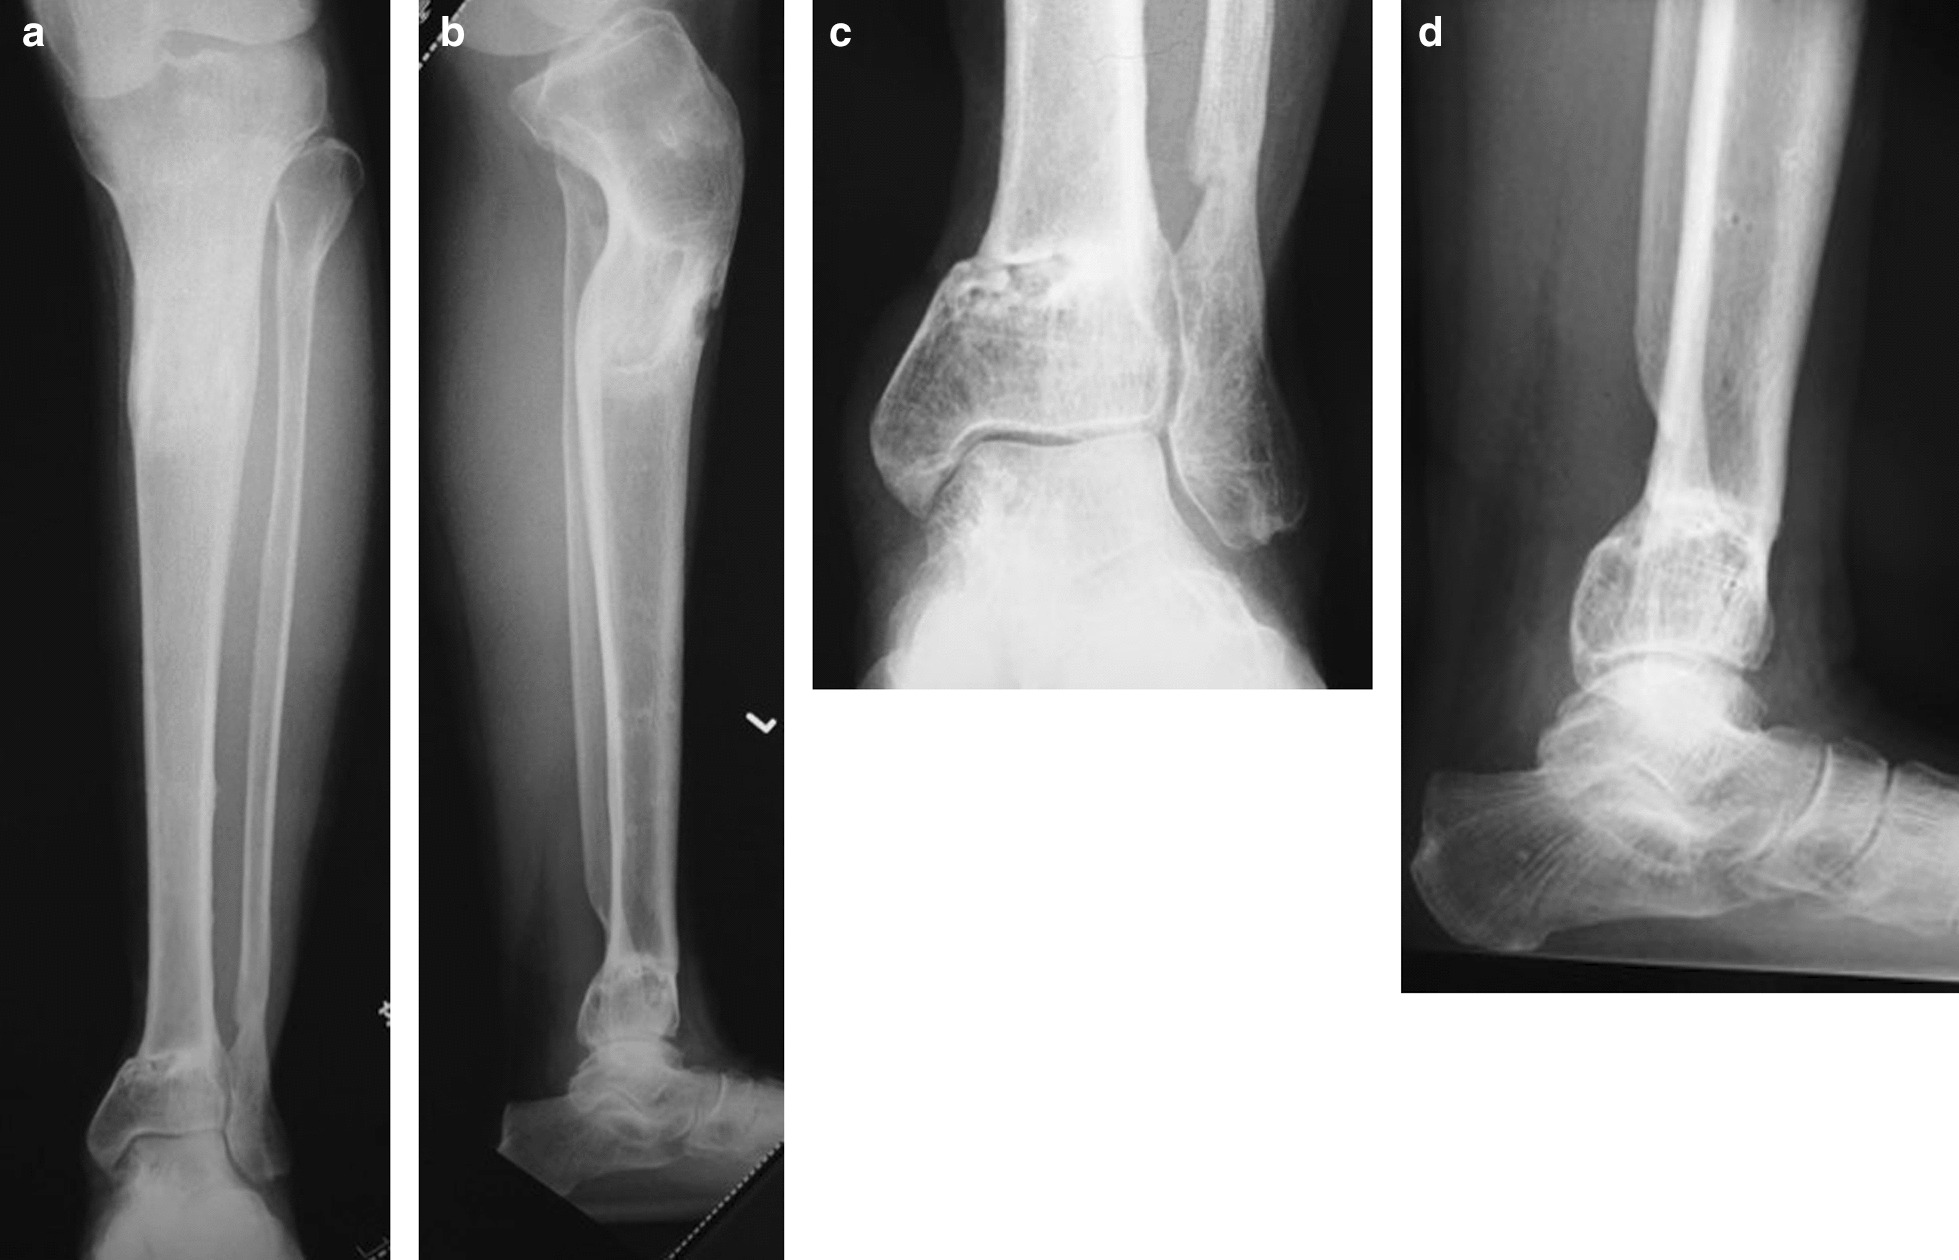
a–d X-ray images taken at postoperative 5-year follow-up
